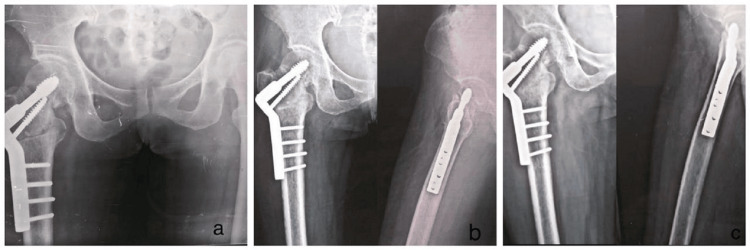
Follow-up radiographs for DHS patient. (a) Immediate postoperative X-ray;
